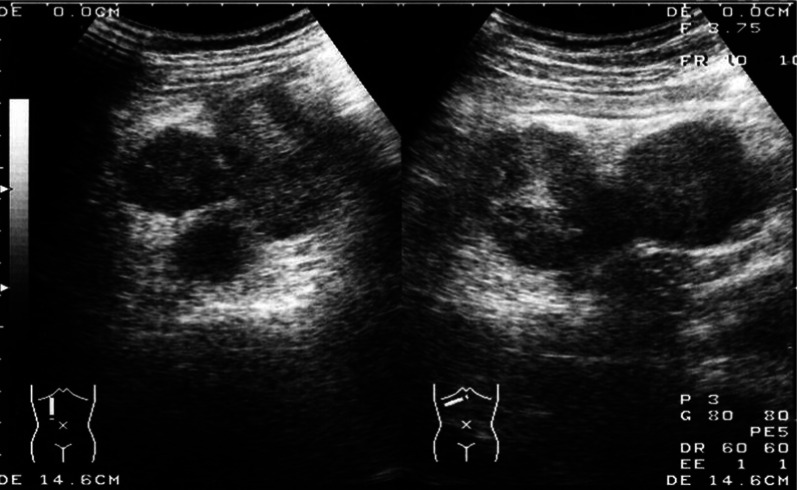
Ultrasonography showing two heterogeneous echogenic round contiguous masses, 5 cm in size each, at the region of the pancreatic head
